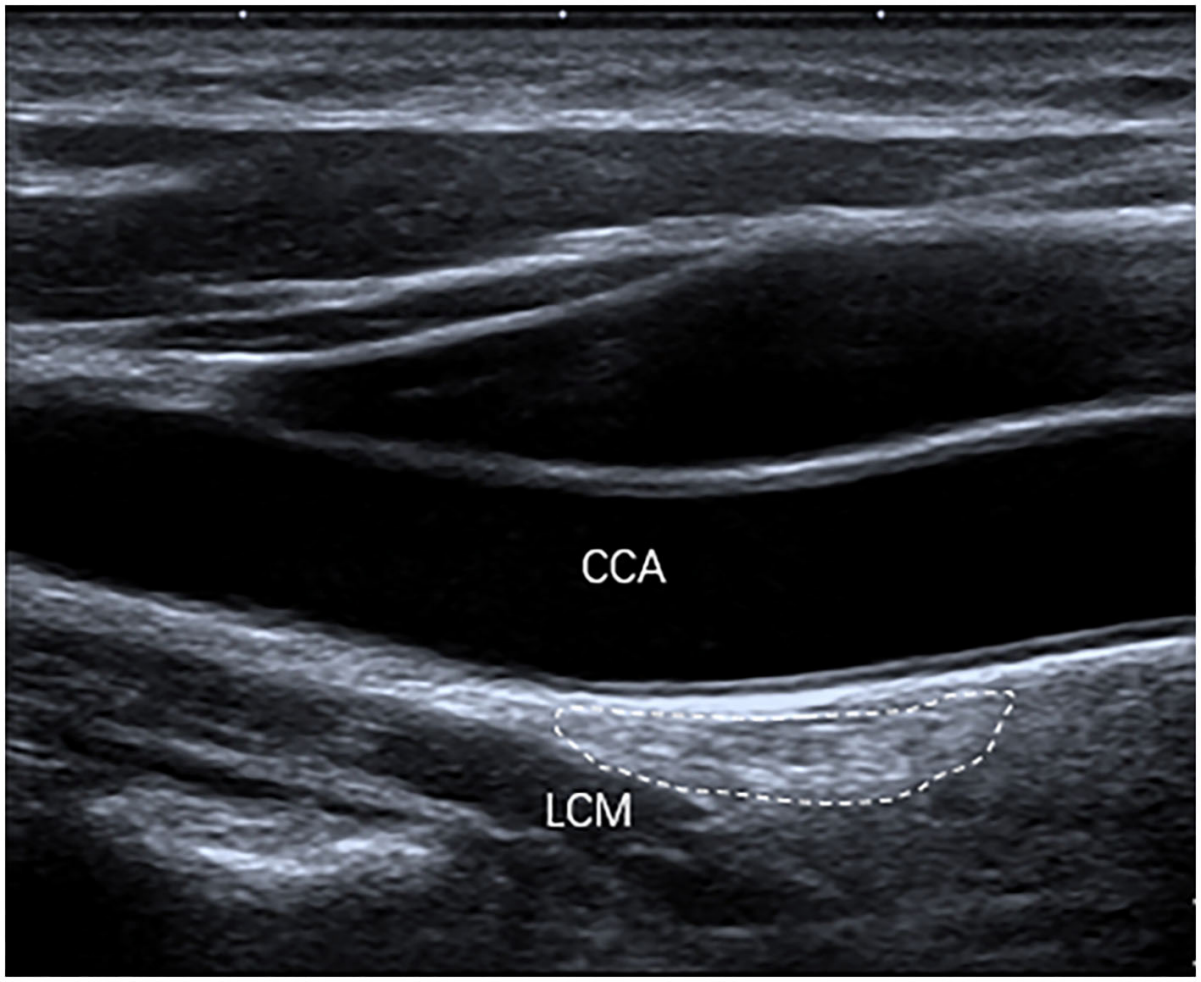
The SG, with an oval shape (outlined) and hyperechogenicity, was located between the common carotid artery (CCA) and the longus colli muscle (LCM) on the longitudinal ultrasound image.
